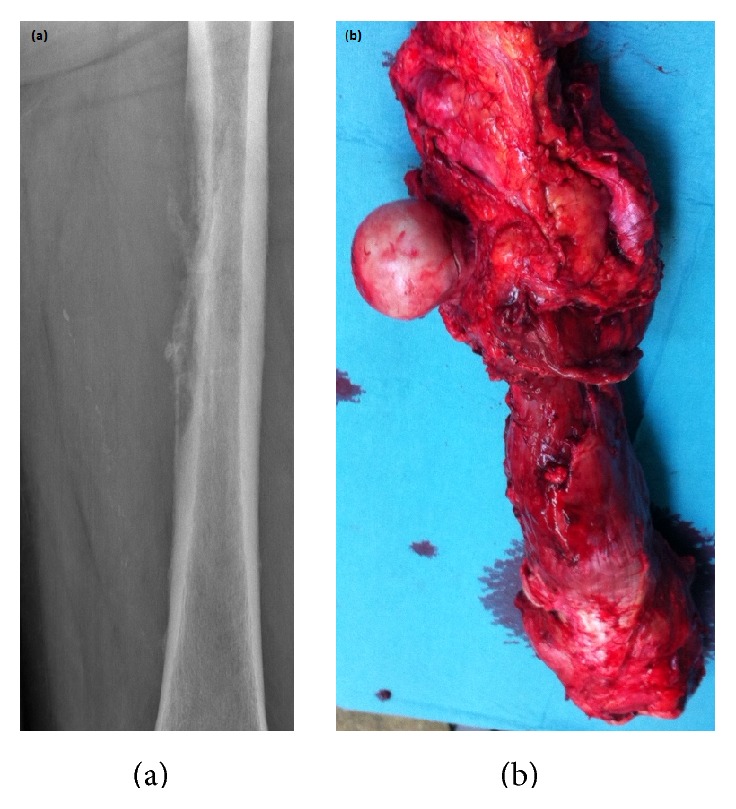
Plain X-ray of the left femur in a patient with penile cancer without any evidence of lymphatic spread reveals a lytic metastasis in the shaft of the bone (a). The left femur excised en bloc prior to reconstruction with a tumor endoprosthesis (b).
